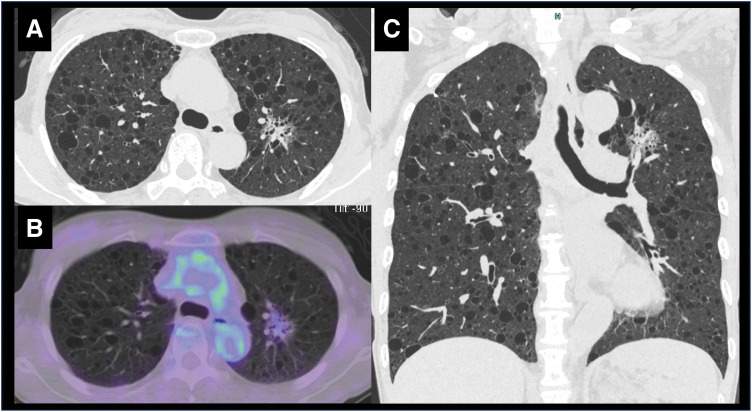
(A) A chest CT scan shows an enlarging part-solid ground-glass opacity within a lung that has numerous thin-walled cysts. (B) PET-CT revealed radiotracer uptake in the nodule, with an SUVmax of 1.39. (C) Considering the tumor’s location, lobectomy was selected as the surgical procedure. SUVmax, maximum standardized uptake value
